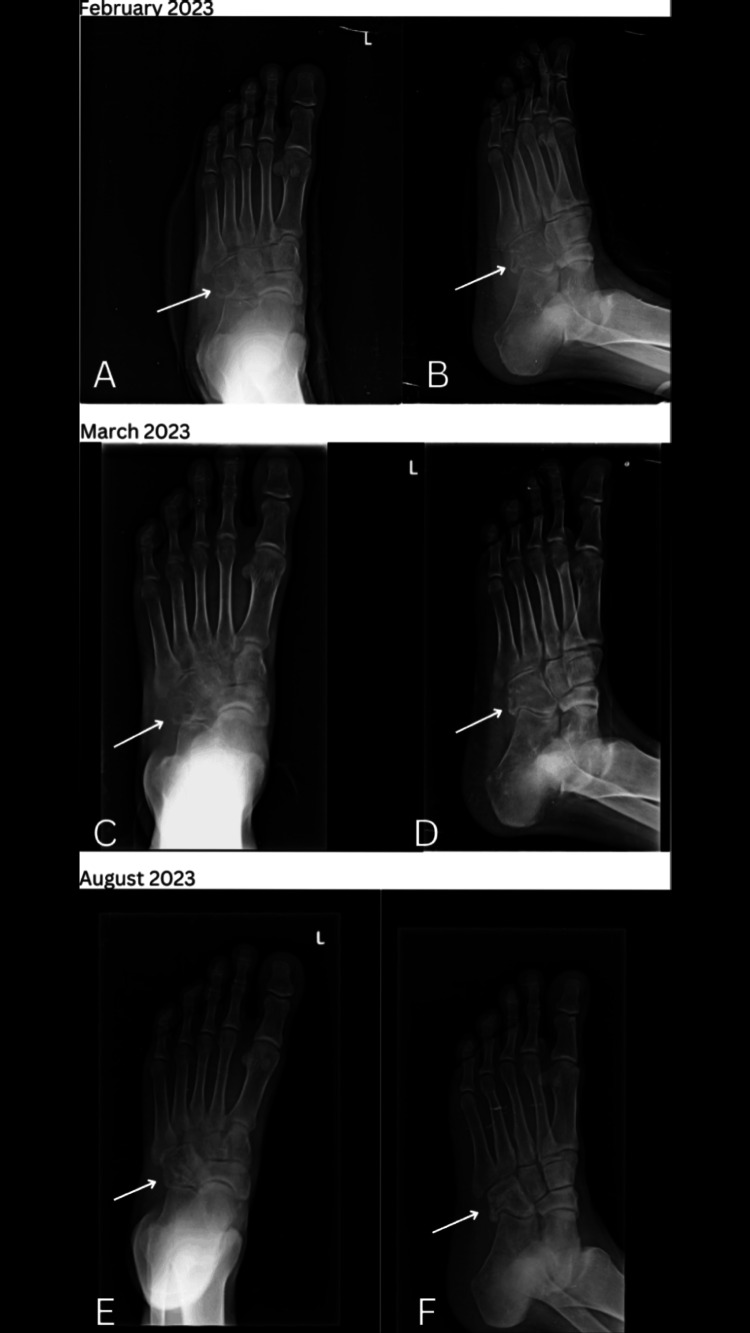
Serial radiographs following the start of anti-tubercular therapy (ATT) showing resolution. A, B: radiograph of cuboid lesion after one month of ATT; C, D: ongoing resolution of cuboid lesion after two months of ATT; E, F: improvement in cuboid lesion after completion of ATT ATT: anti-tubercular therapy
